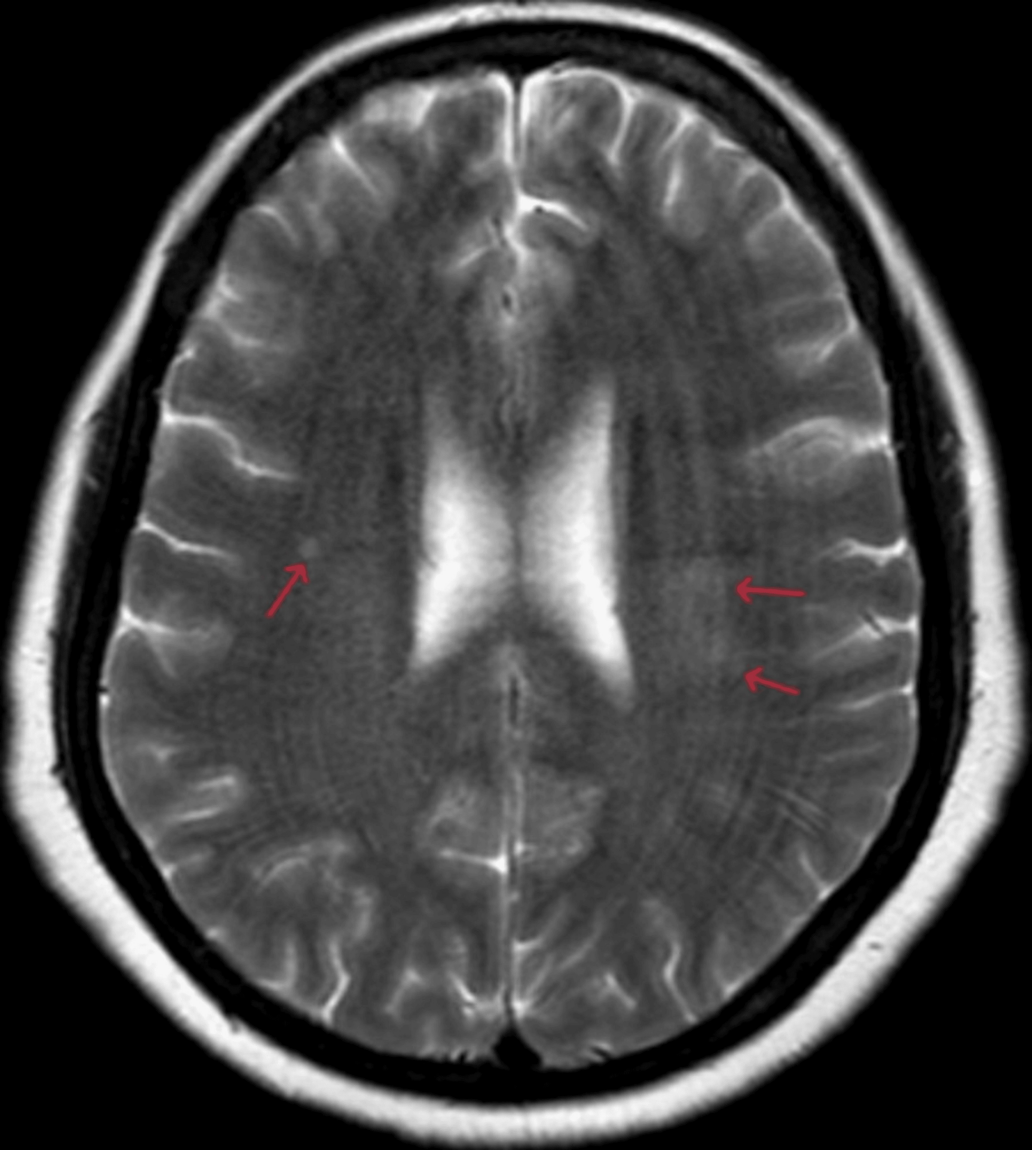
Axial T2-weighted MRI of the brain showing the lesions. Small nodular hyperintensity on the right side and diffuse hyperintense lesions on the left side (arrows).
